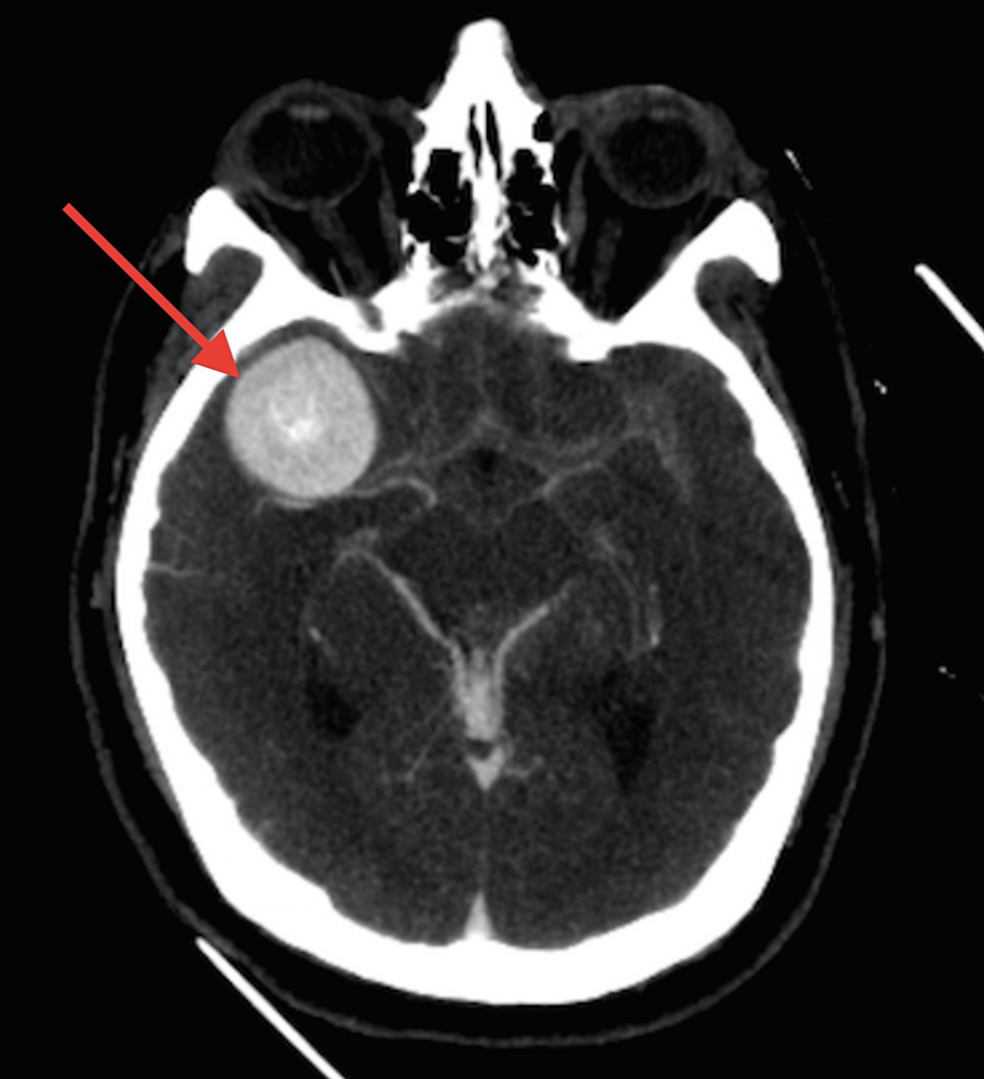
Cerebral CT scan on admission presented a giant aneurysm of the right middle cerebral artery with extensive and diffuse subarachnoid hemorrhage and intraventricular hemorrhage.
